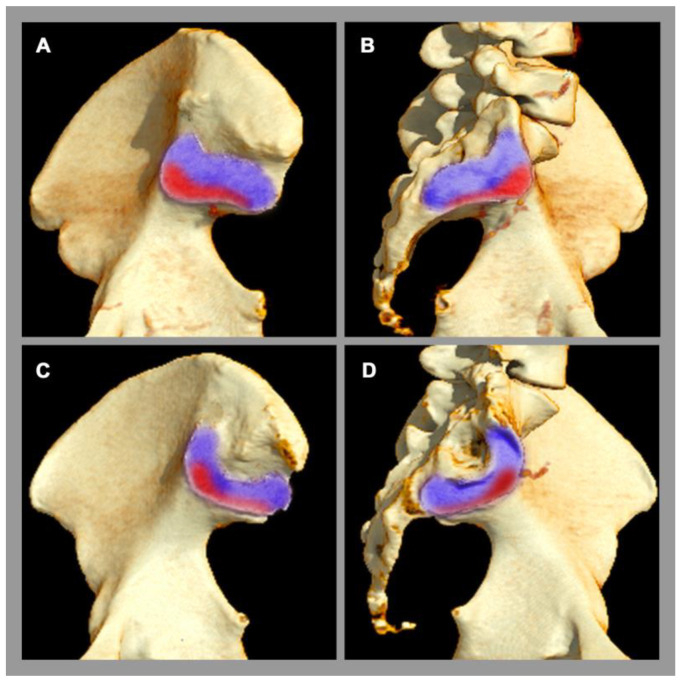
Sacroiliac joint load zones. 3D computed tomography reconstruction. (A,C) Lateral view of the ilium. (B,D) Lateral view of the sacrum. Male pelvis (A,B). Female pelvis (C,D). There are sex differences in the distribution of the load zone (red area) with respect to the rest of the joint surface (purple area). In women, the center of gravity is more ventrally located.
